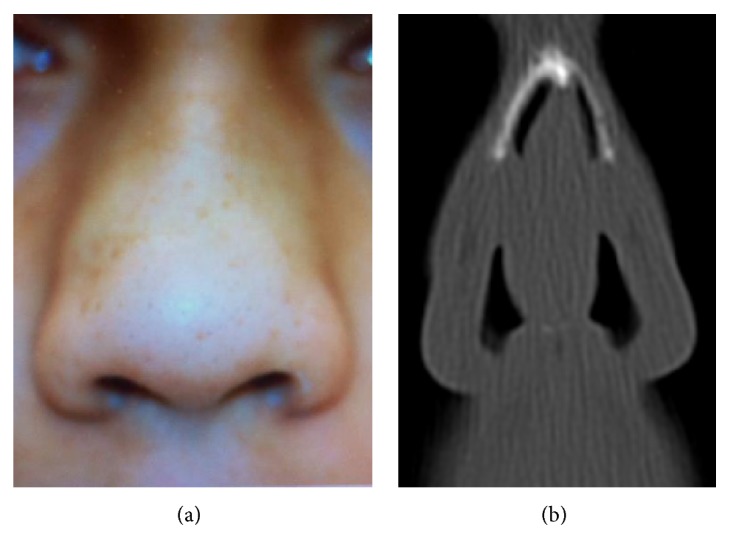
(a) Frontal view of the external nose. Note the swelling of the lateral walls. (b) A CT scan of the anterior nose. Note the wide nasal septum touching the swellings of the lateral pseudotumors which together cause a bulging of the lateral walls.
